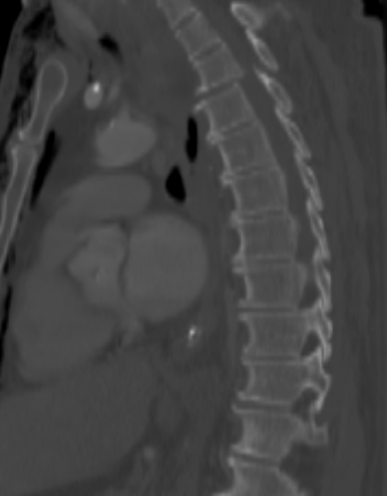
Sagittal reconstructions of chest CT scan demonstrated a T3-T4 subluxation indicative of a DISH hyper-extension-type fracture.
